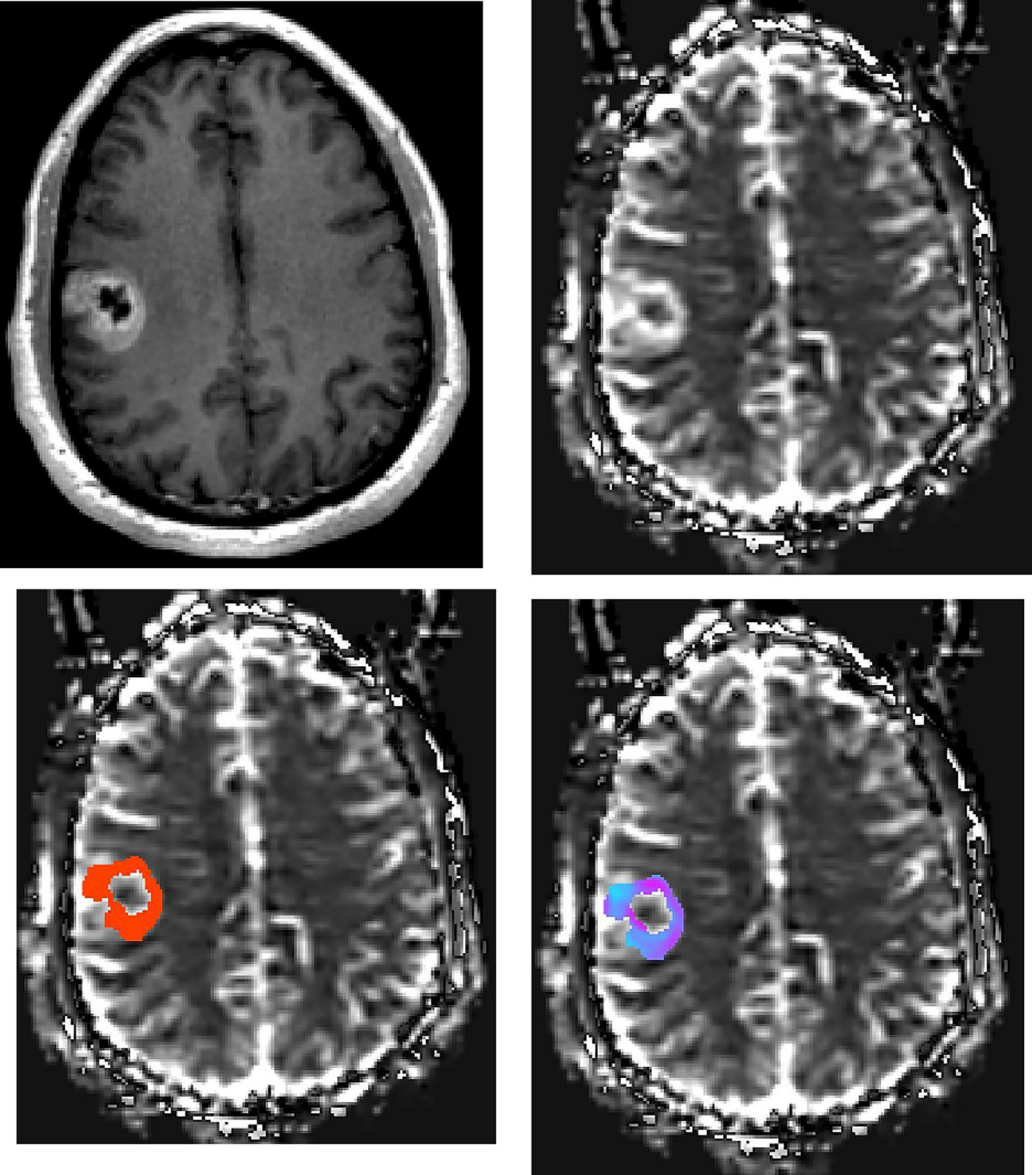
Patient with GBM. Axial (a) contrast-enhanced T1-weighted image shows the right frontal GBM. rCBV maps at the same axial level show (b) lesion with high perfusion in solid component, (c) manually drawn region of interest including contrast-enhanced tumor avoiding any cystic necrotic part to measure rCBV, and (d) standard deviation (SD) map of rCBV reflecting the variation of lesion rCBV. The variety of colors in SD map (d) represent the differences in rCBV values, where pink represents a higher value and blue represents a lower value.
